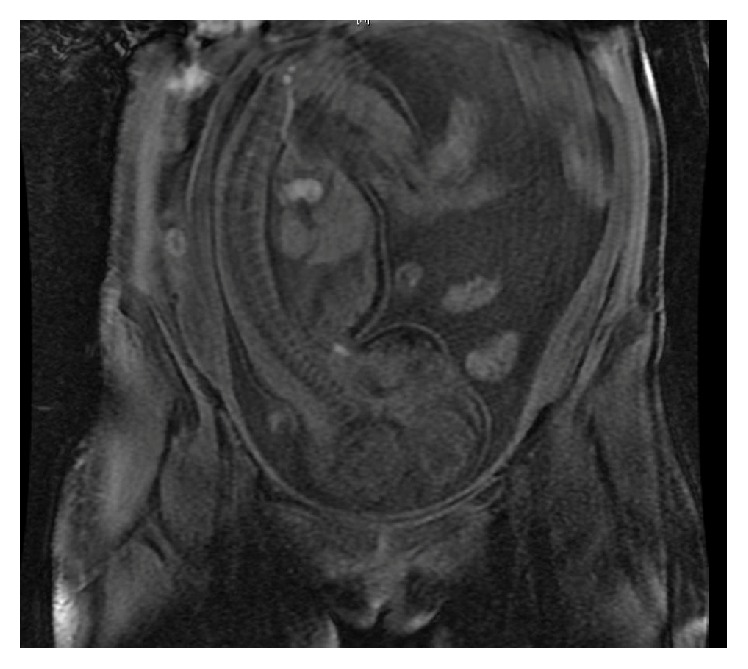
MRI showing coronal view of resolution of right iliacus muscle abscess following percutaneous drainage and antibiotics during pregnancy.
